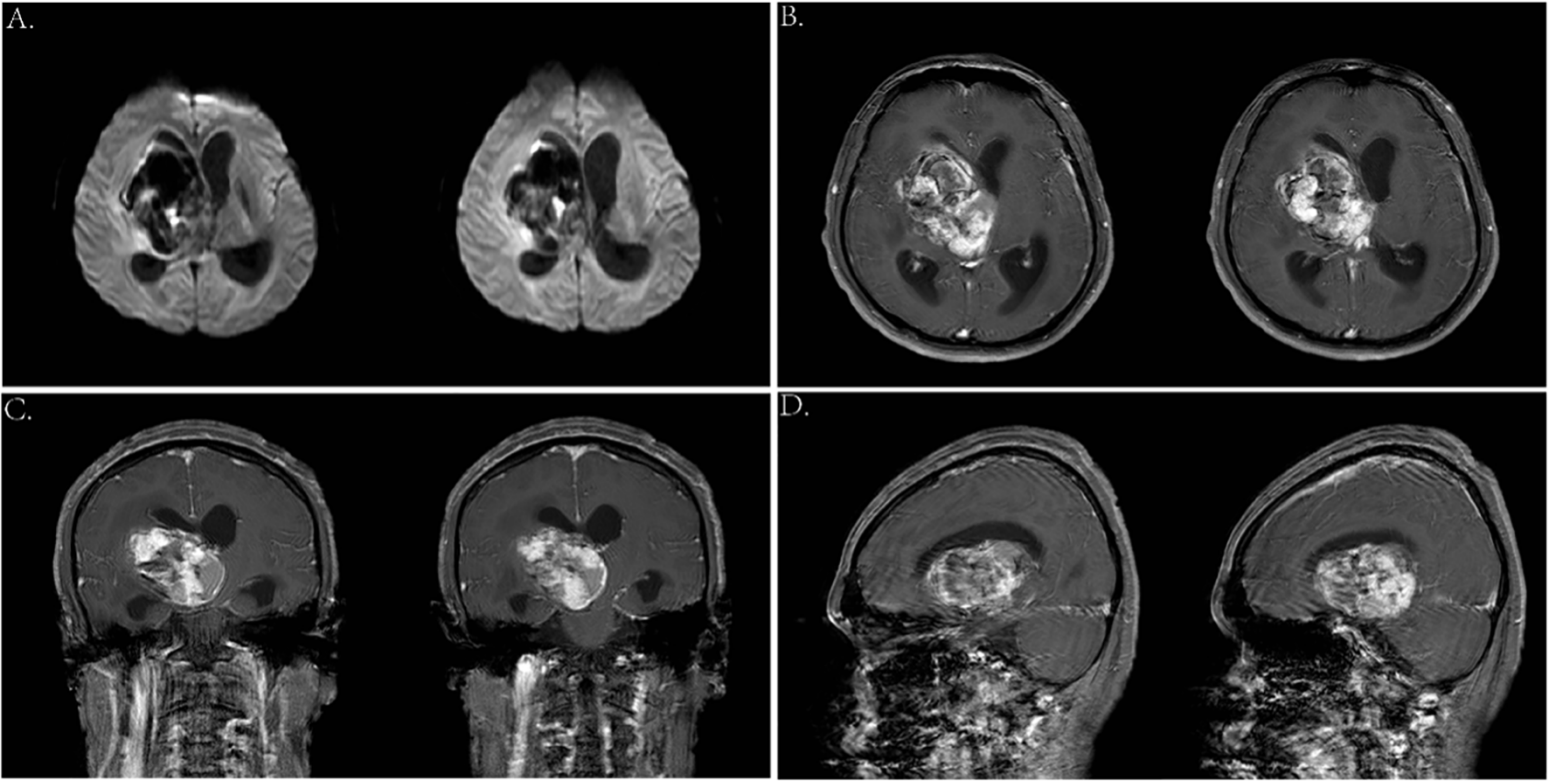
(A) Axial DWI images. (B—D) Enhanced MRI scan showed a 6.4 * 4.8 * 5.1-cm mass in the right basal ganglia.
